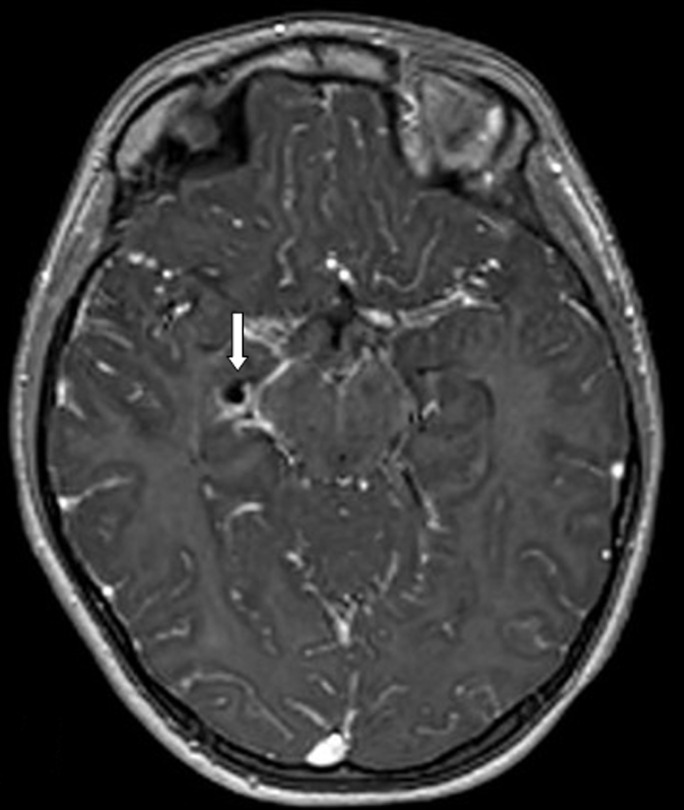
axial MRI T1 after injection of gadolinium: cystic lesion without peripheral enhancement (arrow)
